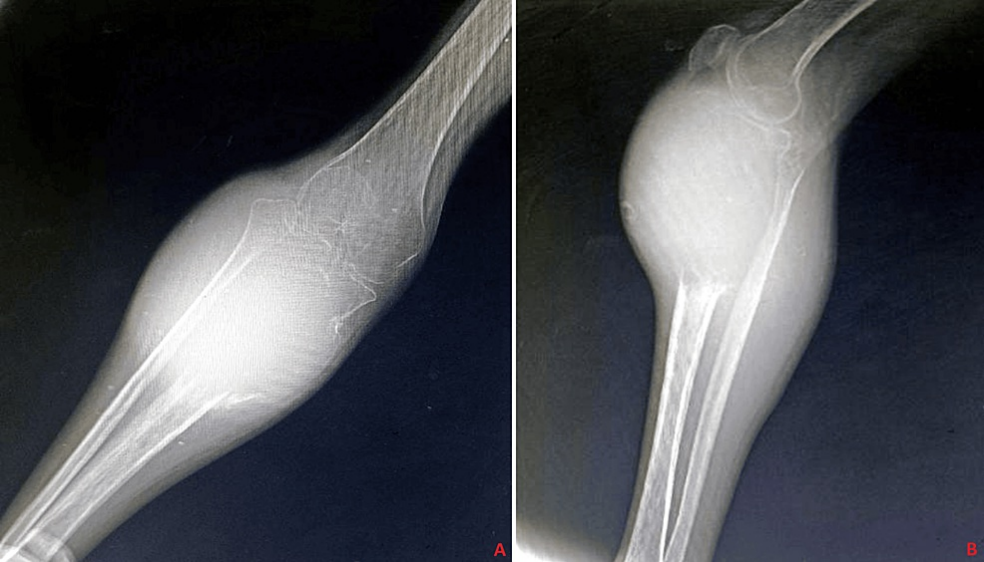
(A-B) X-ray of the right knee with tibia suggestive of osteosclerotic lesion with ill-defined margin over the proximal tibia and periosteal reaction present
